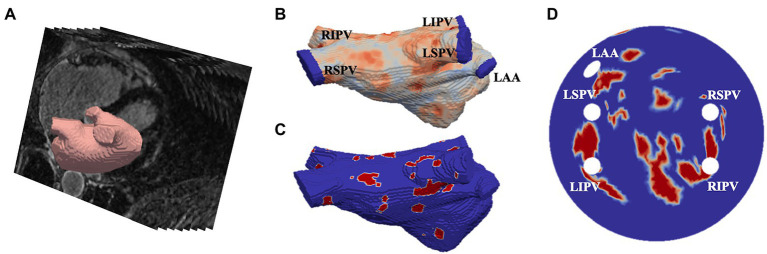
Generating image-based 2D left atrial tissues. (A) LGE-MR image (greyscale) with segmented LA (red). (B) The 3D LA with LGE-MRI intensity distribution and the PVs and LA appendage (LAA) clipped. (C) The thresholded 3D LA with fibrosis in red and healthy tissue in blue. (D) The LA unwrapped onto a standardised 2D disk, with fixed four PVs and the LAA and fibrotic areas mapped. PVs and LAA are labelled in (B,D).
